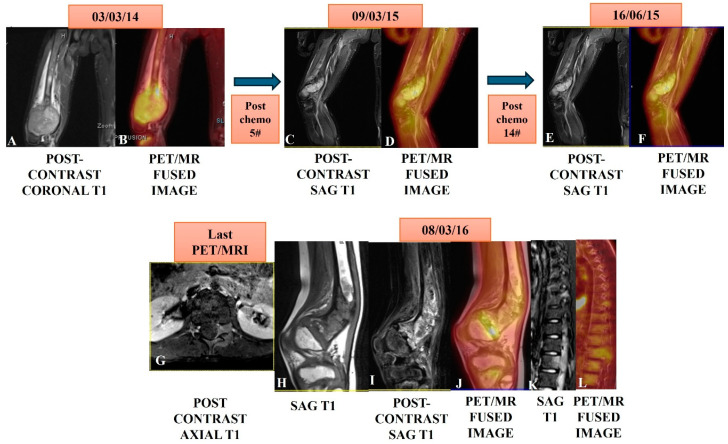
A case of osteosarcoma of the distal right femur in an eight-year-old male child showed an expansile enhancing and metabolically active mass in the distal femur (A,B) in a PET/MRI conducted on 03/03/14. The patient underwent serial PET/MRI studies after five cycles on 09/05/15 and then, after 14 cycles of chemotherapy on 16/06/15, showed marked regression in bulk and near complete metabolic regression in serial scans (C,D,E,F). A follow-up PET/MRI scan was taken after nine months on 08/03/16 and showed residual/recurrent enhancing (H,I) and a metabolically avid marrow lesion (J) in the meta-diaphysis of the distal femur. Note: the [^18^F]-FDG avid marrow lesion left posterior elements of D9 and L1 (K,L) are suggestive of metastasis with left spinal epidural soft issue extension at the L1 level (G).
